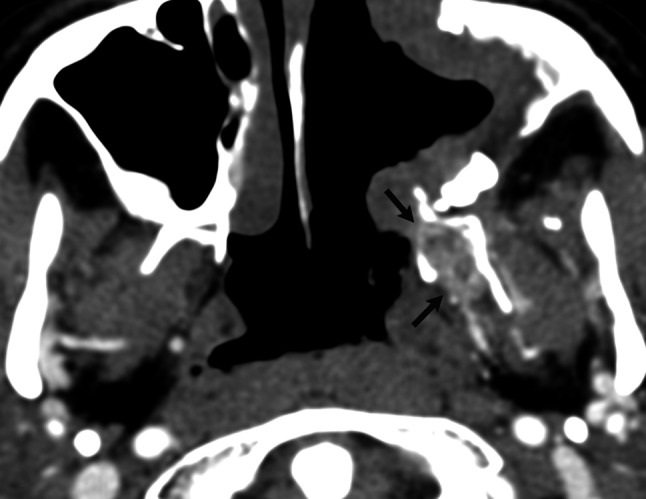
Axial CT scan after application of contrast medium shows small, submucosal residual tumor between the pterygoid plates (*arrows*)
